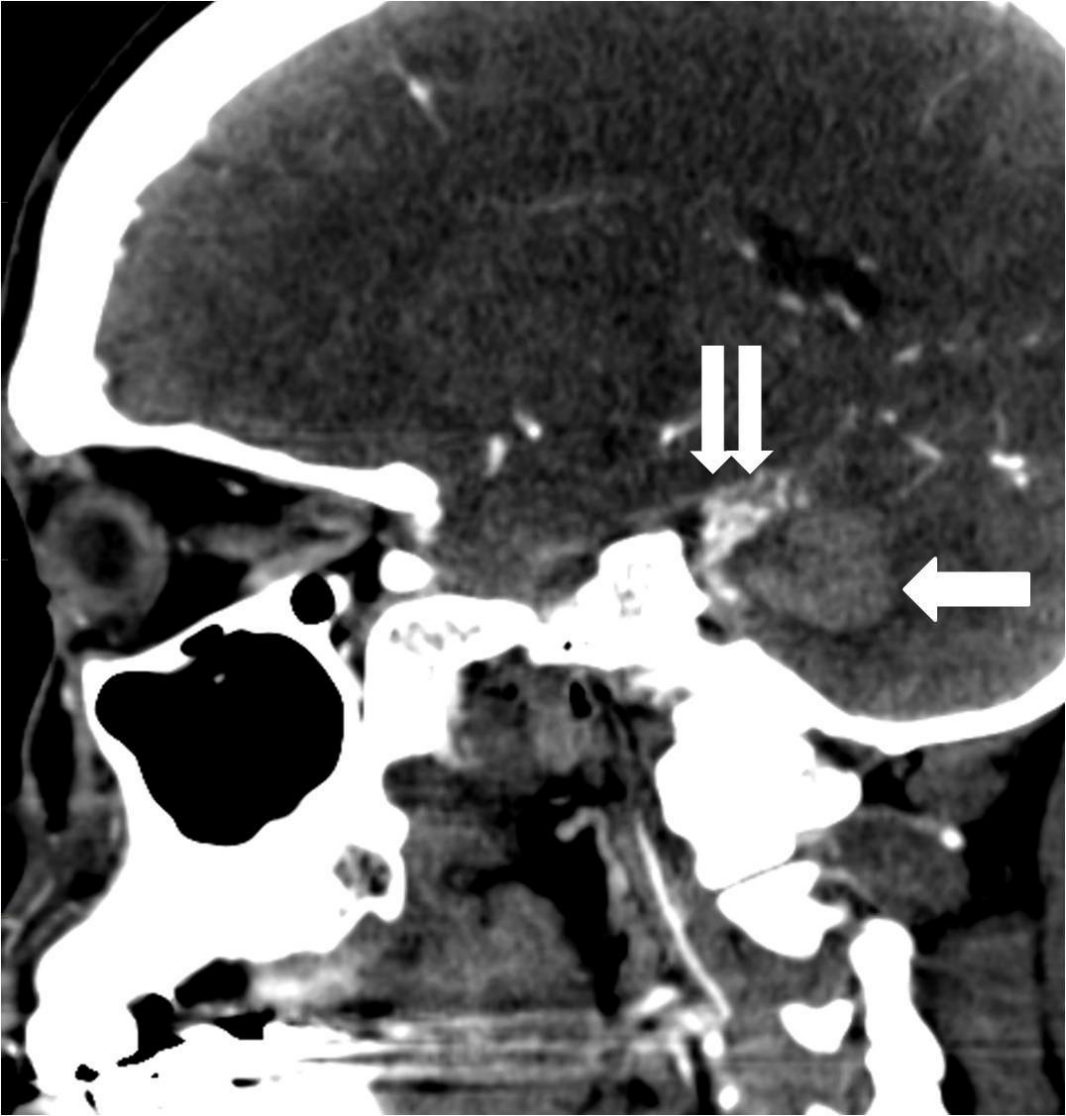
Sagittal reconstruction image from a computed tomography angiogram demonstrates the cerebellar parenchymal hematoma (arrow) and the adjacent arteriovenous malformation (double arrow).
